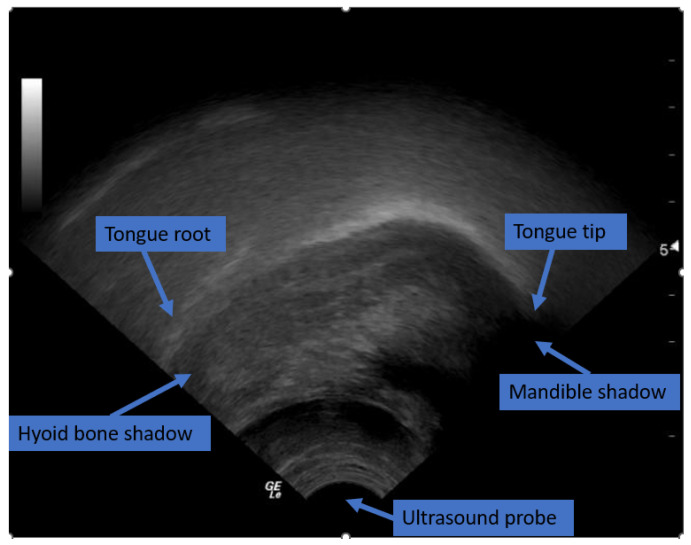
Ultrasound image of the tongue showing the tongue tip and root in the sagittal plane. The ultrasound probe on the bottom and the shadowing effect of the mandible and hyoid bone are visualized. The copyright for this ultrasound picture belongs to the author of this article, Khalid Al-hammuri [5].
